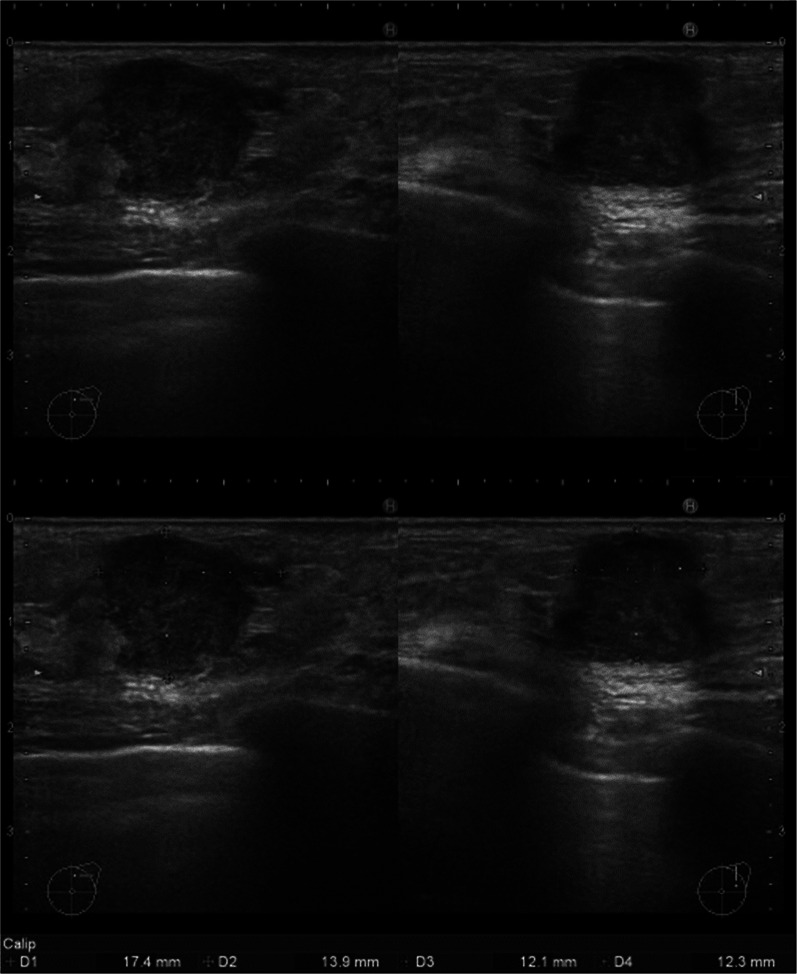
Ultrasound showing a 17.4 × 13.9 × 12.1-mm lobulated mass in the left breast
